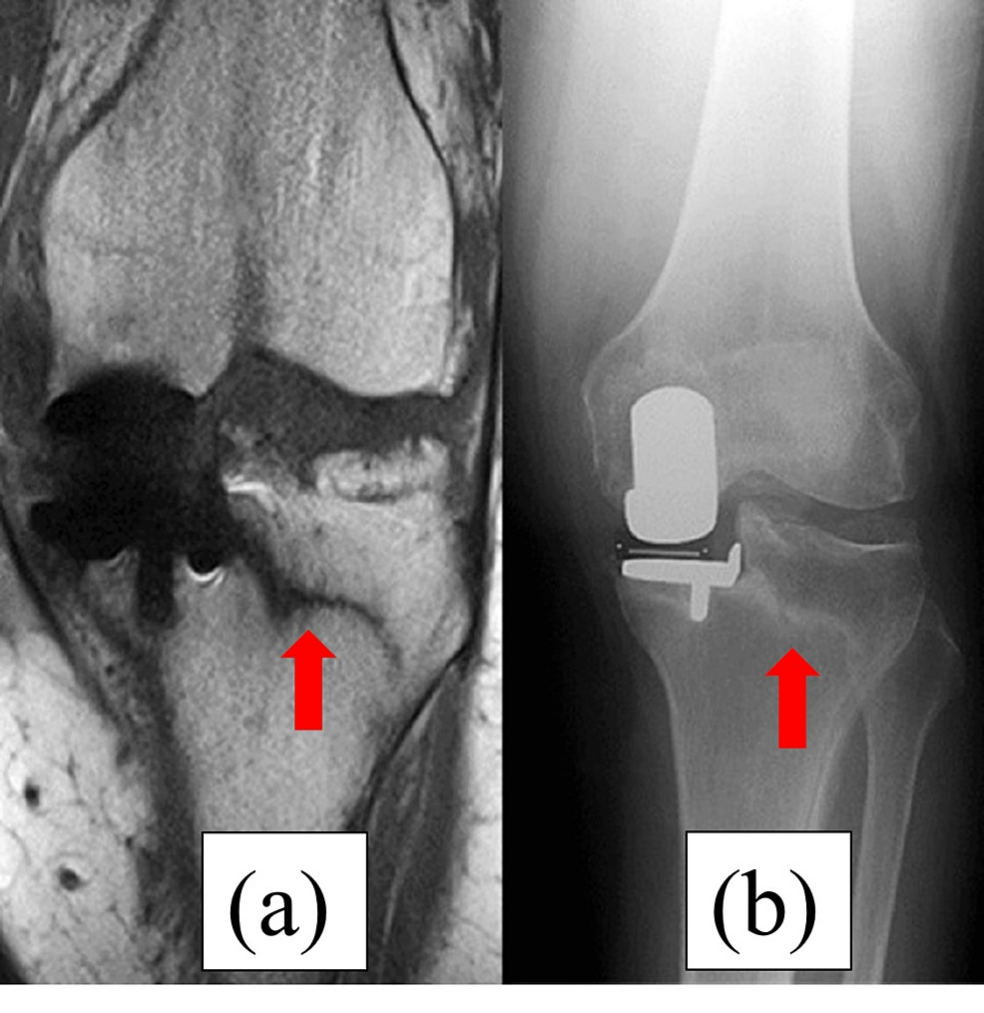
MRI and radiography at five weeks postoperatively. (a) The red arrow of the MRI shows the same fracture line as Case 1. (b) The red arrow of radiography shows the same fracture line as the MRI.
